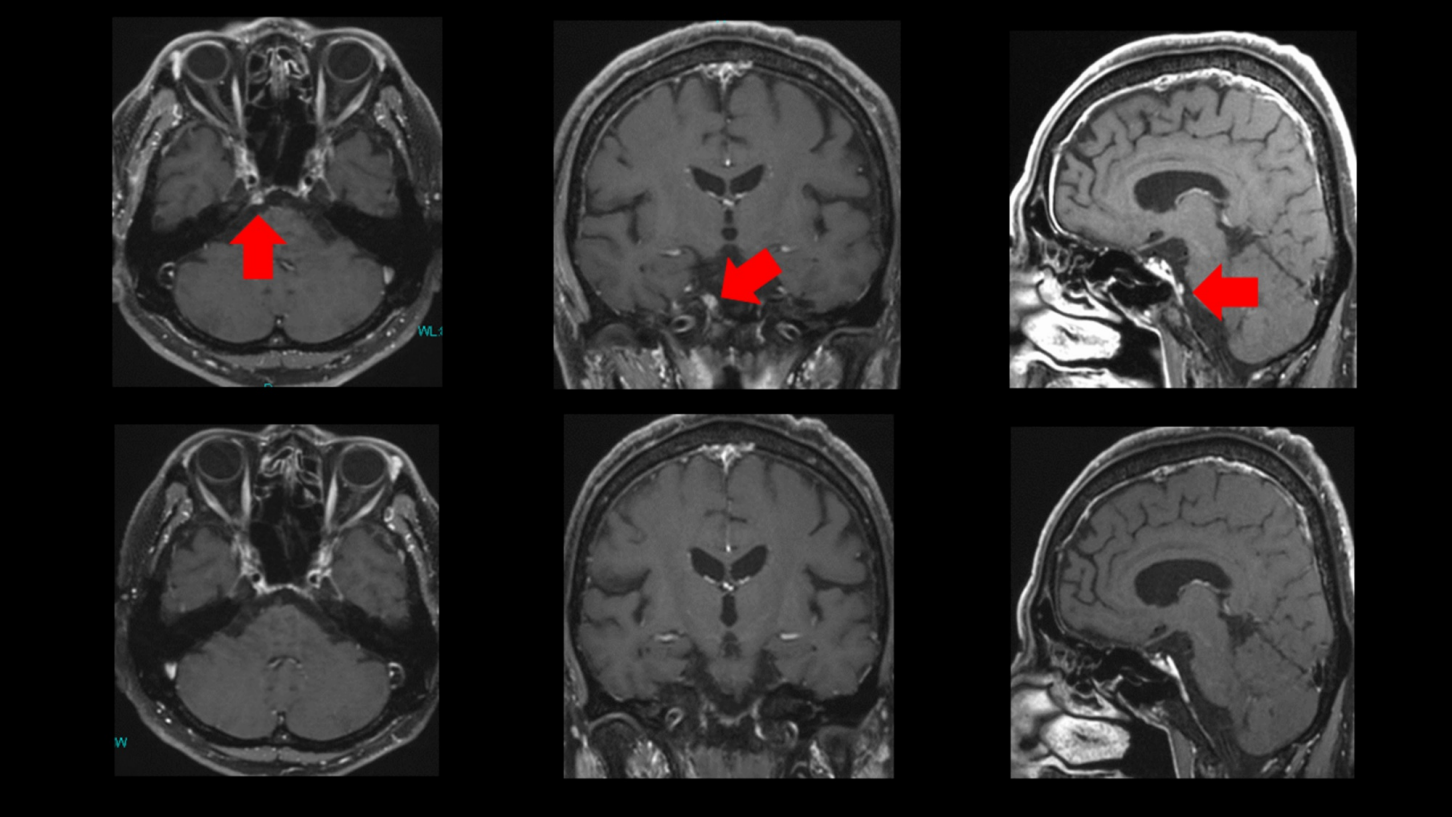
Contrast-enhanced MRI of Case 7 Contrast-enhanced MRI before CyberKnife (top) and after CyberKnife (bottom). The tumor located in Dorello's canal (red arrow) disappeared after treatment. MRI: magnetic resonance imaging
